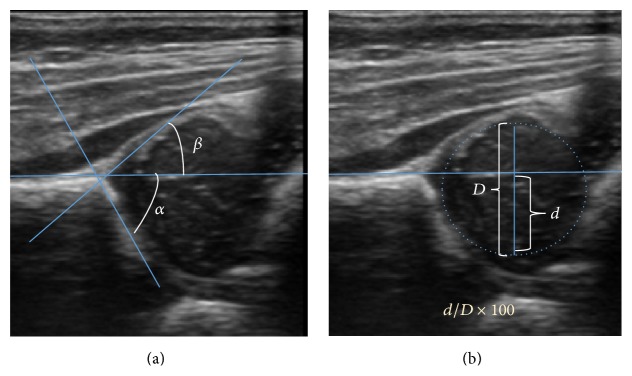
(a) Useful ultrasound measures in neonatal hip sonography, alpha and beta angles. (b) Measurement of femoral head coverage.
